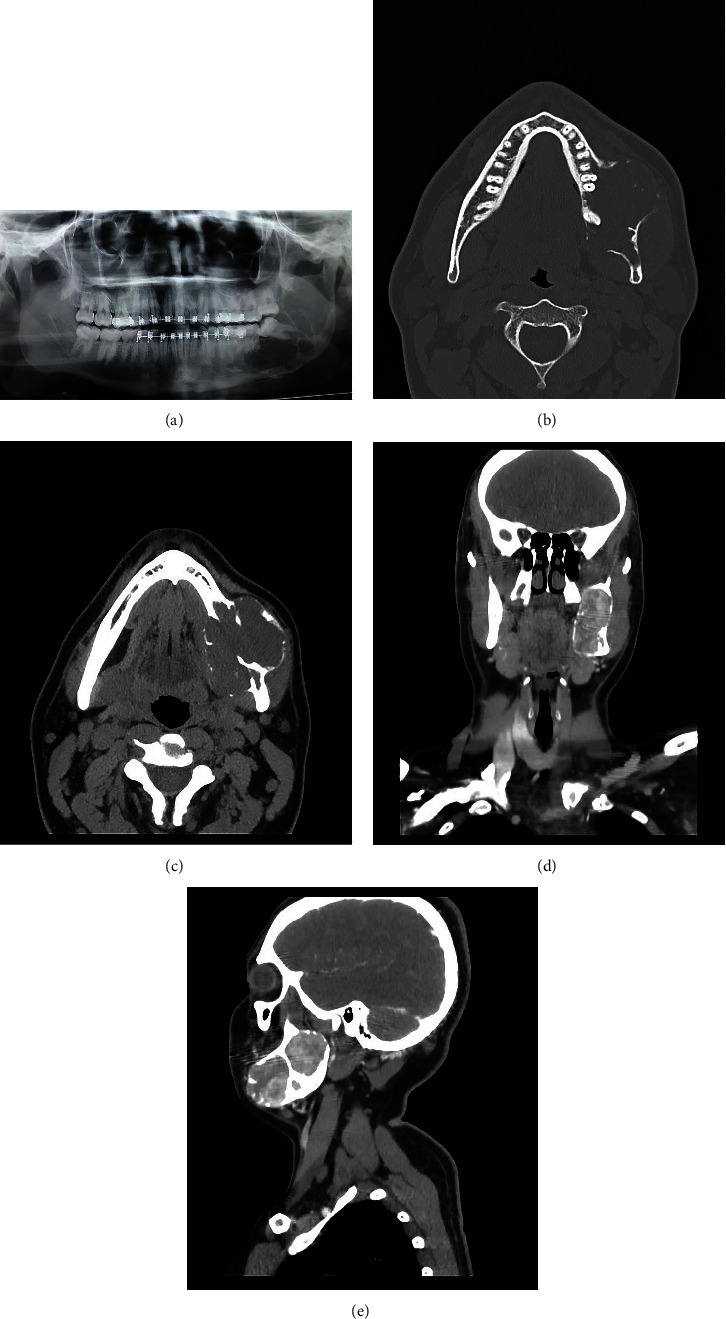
Panoramic radiograph revealed a multilocular radiolucency in the body and ramus of the mandible (a). Computed axial tomography revealed a large hypodense lesion exhibiting cortical bone destruction (b–e).
